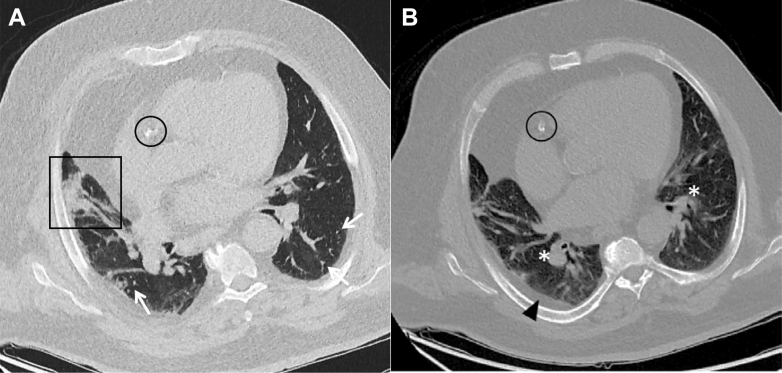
A, B, Chest CT scan on admission shows A, a focal area of subsegmental consolidation with visible air bronchogram in the middle lobe (boxed area) compatible with beginning bronchopneumonia. Furthermore, nodular hyperdensities (arrows) in the peripheral lung parenchyma are visible, potentially reflecting vascular calcifications. B, Scan additionally shows signs of pulmonary venous congestion with enlarged diameter of pulmonary veins in comparison to adjacent bronchus on both sides (asterisk) and a right-sided pleural fat deposition dorsobasal (closed triangle). Both images show minimal coronary calcification (circled area).
